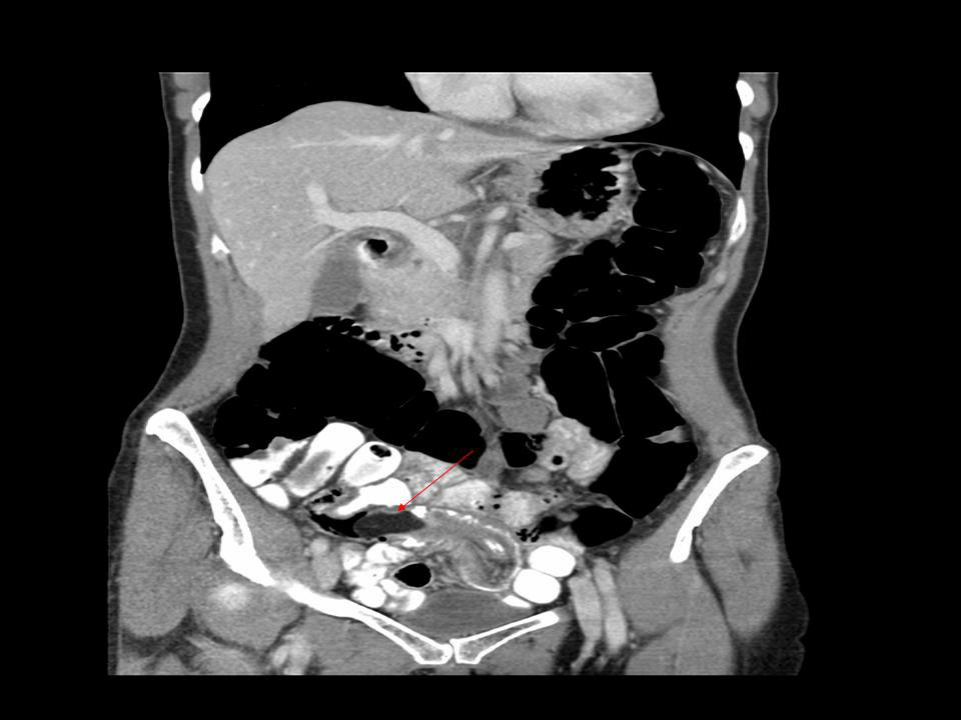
Contrast-enhanced computed tomography scan of the abdomen demonstrates a smooth-walled, low-density intramural lesion. It measures 3.2 × 1.6 cm. The ileum at the proximal end of the lesion is mildly dilated with a centrally placed narrowed channel of contrast consistent with an intussusception.
